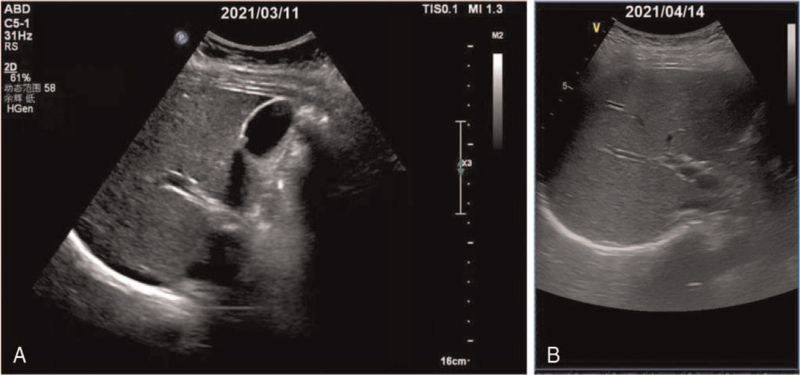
The ultrasound of the liver on March 11, 2021 (A) and April 14, 2021 (B). There was no dilatation of the intrahepatic or extrahepatic bile ducts and hepatomegaly or splenomegaly appeared in 2 images.
